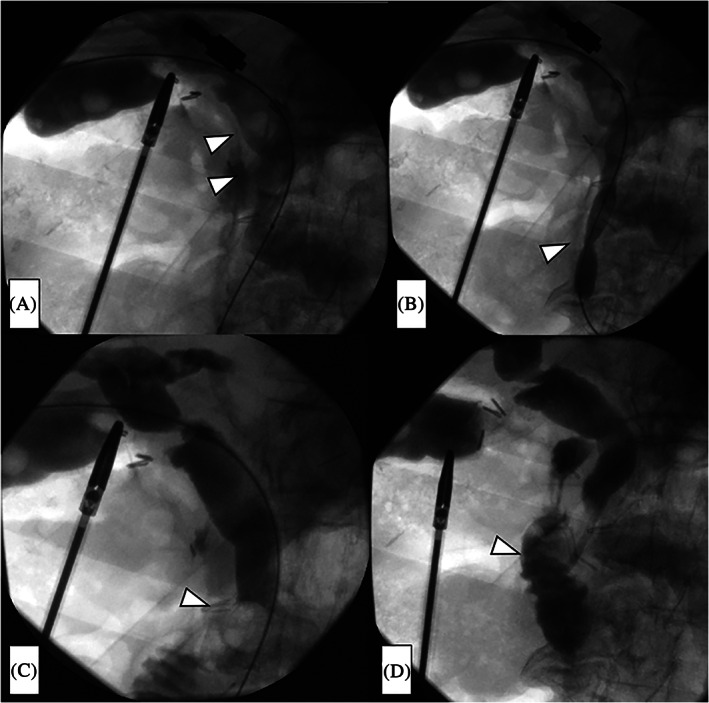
Radiographic images during one‐stage laparoscopic transcystic papillary balloon dilation and laparoscopic cholecystectomy. A, The presence of choledocholithiasis was confirmed by injecting contrast medium into the bile duct from the sheath (arrowheads). B, Balloon dilation of the papilla of Vater (arrowhead). C, The stones were extruded using the balloon (arrowhead), and the common bile duct was cleaned. D, The absence of any residual stones was confirmed, and a pigtail‐shaped drainage catheter (arrowhead) was inserted to help prevent edema of the papilla of Vater and pancreatitis
